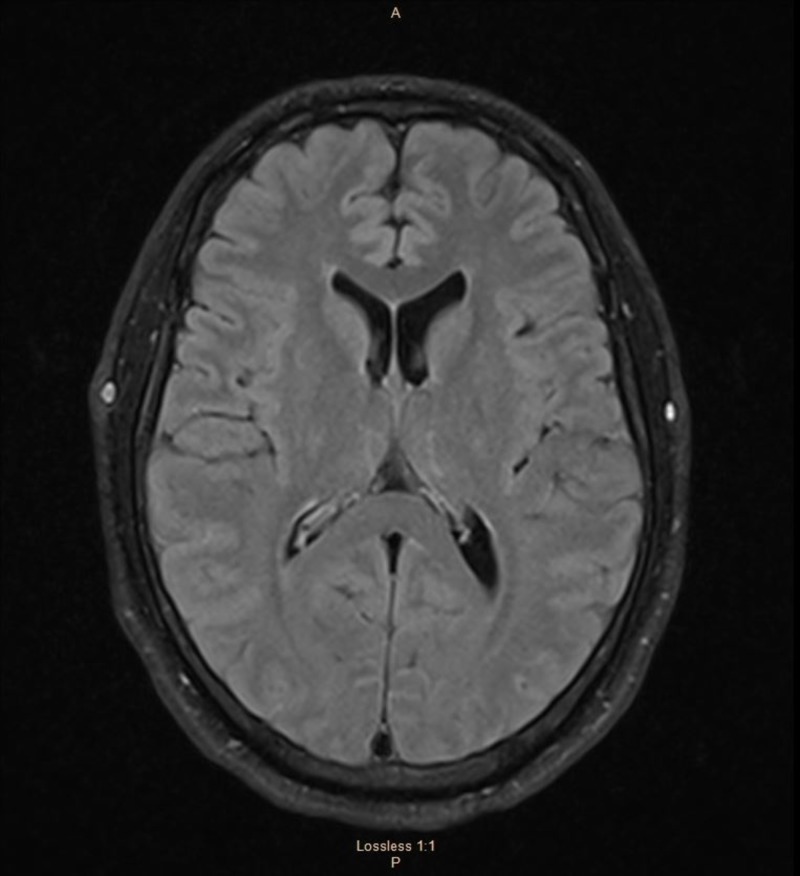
MRI of the brain FLAIR sequence; axial images within normal limits There is no evidence of traumatic brain injury or bullet wound injury, as reported by the patient. MRI: magnetic resonance imaging; FLAIR: fluid-attenuated inversion recovery
